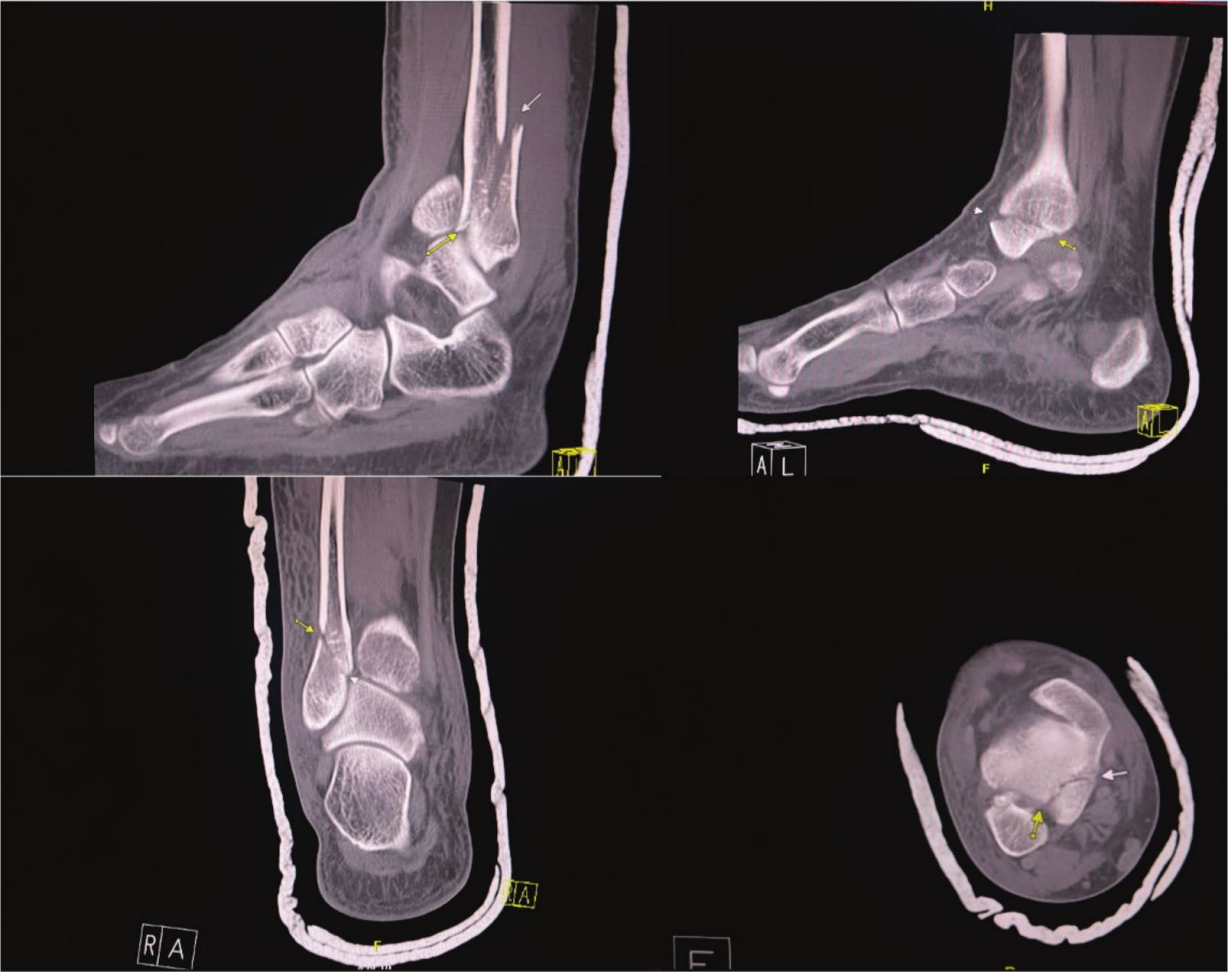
CT scan of the right lower extremity showing a trimalleolar fracture affecting the medial and posterior tibia and lateral fibula (yellow arrows). Image taken post‐manual reduction of ankle joint dislocation and plaster cast application in the emergency department.
